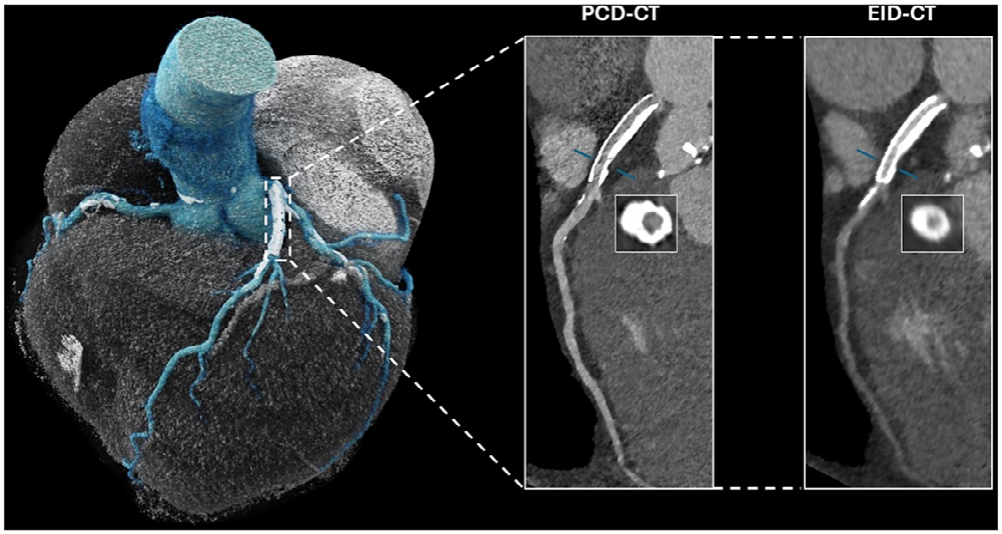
Stent along the left anterior descending artery in a 76‐year‐old male. Reduced blooming artifacts in the photon‐counting detector (PCD)‐CT image (acquired using the ultrahigh‐resolution detector mode and reconstructed to a slice thickness of 0.2 mm) compared to the energy‐integrating detector (EID)‐CT allows better in‐stent lumen visualization.
